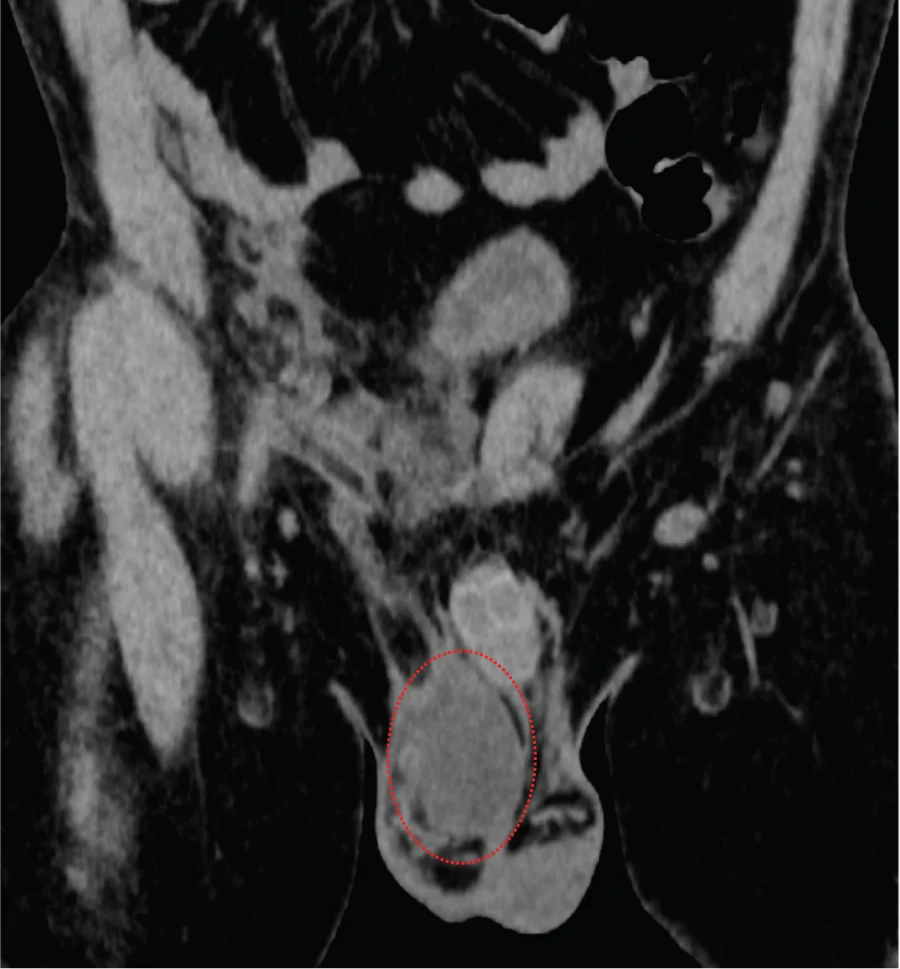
CT on POD 10 revealed a small amount of serous fluid accumulation. A serous fluid collection measuring 2 cm × 2 cm × 3 cm was observed in the right hemiscrotum, as indicated by the red dotted circle.
